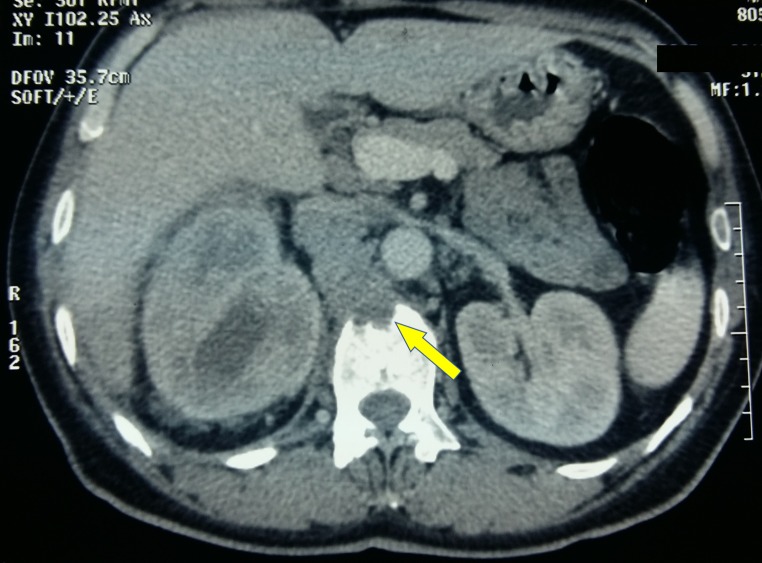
Para-aortic lymph node mass Axial CT scan showing a para-aortic lymph node mass that invaded the L1 vertebral body. CT, computed tomography.
